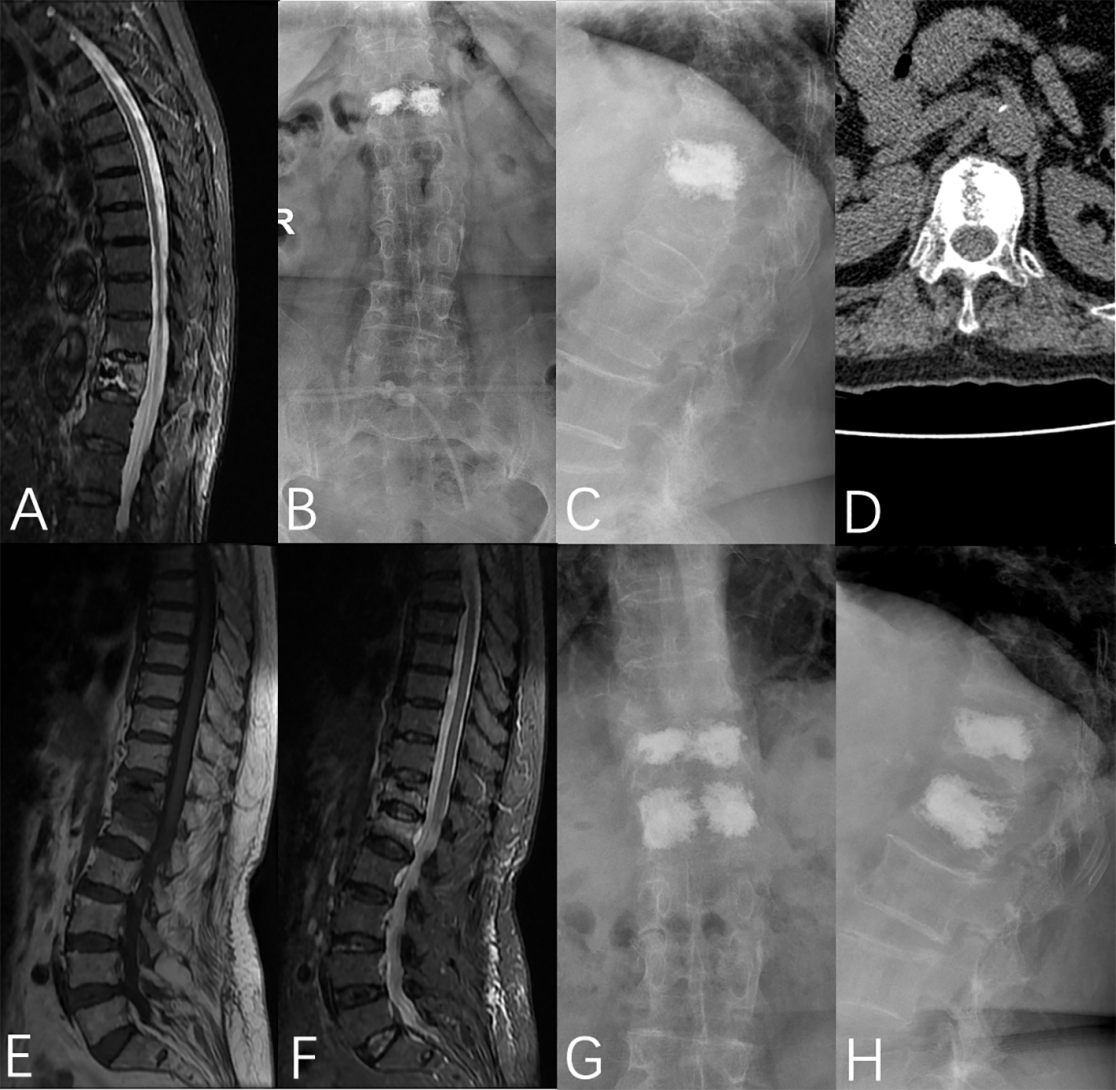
A 76-year-old female was admitted to the hospital with no obvious cause of low back pain for 1 week, was diagnosed with OVCF (T12), and underwent PVP under local anesthesia on the third day after admission. (A) Preoperative fat-suppressed image showed T12 vertebral fracture, (B, C) T12 vertebral bone cement filling could be seen in the anterior and lateral X-rays after the operation, (D) Postoperative CT showed that the bone cement was unevenly distributed on the bilateral sides of the vertebrae, (E, F) MRI showed L1 vertebral fracture 5 months after the operation, (G, H) T12 and L1 vertebral bodies were filled with bone cement in the anterior and lateral X-rays after the operation.
